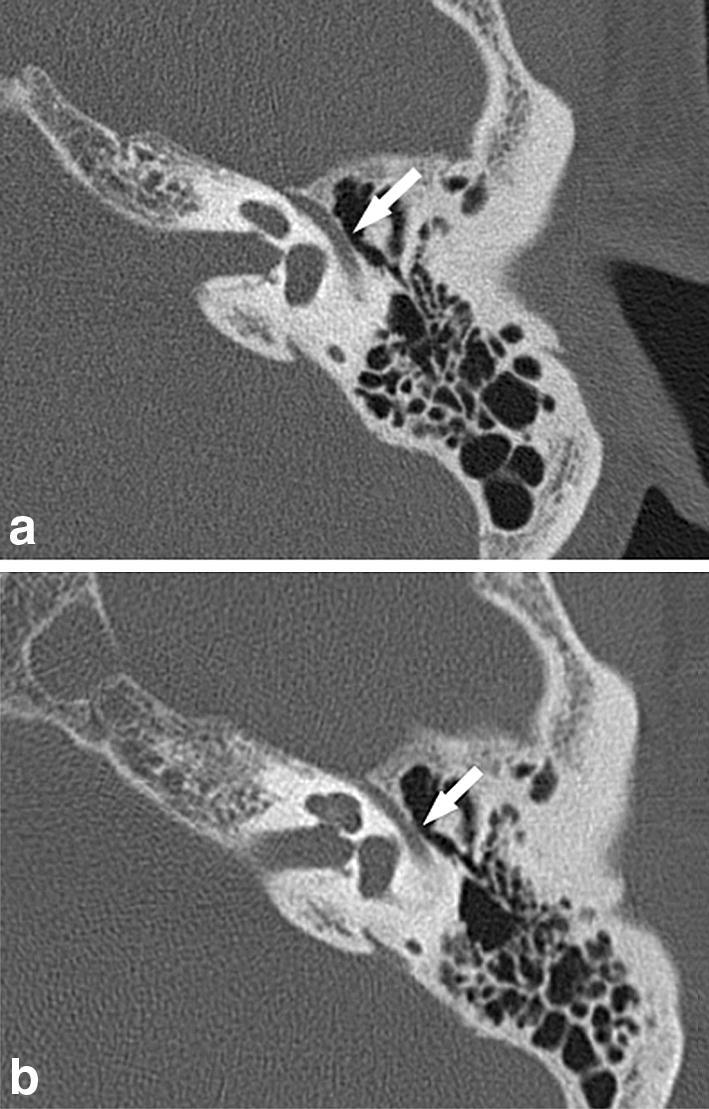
Axial images of the left temporal bone CT from the same case. Image quality scores for the tympanic segment of the facial nerve canal (arrow) by Reviewers 1 and 2 are five and four, respectively, on UHRCT (a), and three on MDCT (b) by both Reviewers. By both Reviewers, dehiscence in this anatomy was considered to be present on MDCT but not on UHRCT
